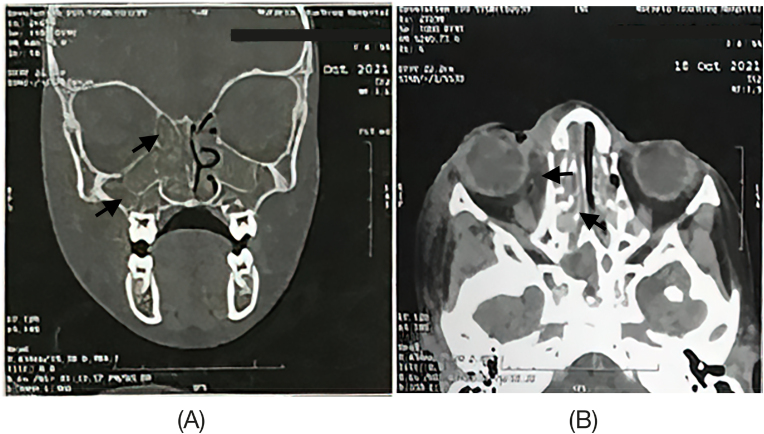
Non-contrast CT scans of the paranasal sinuses and orbit on day +2. (A) Coronal view showing complete obliteration of the left and right maxillary and ethmoidal sinuses by mucosal thickening and retained secretions with obliterated osteomeatal units. (B) Axial view showing right preseptal and peri-orbital soft tissue swelling.
